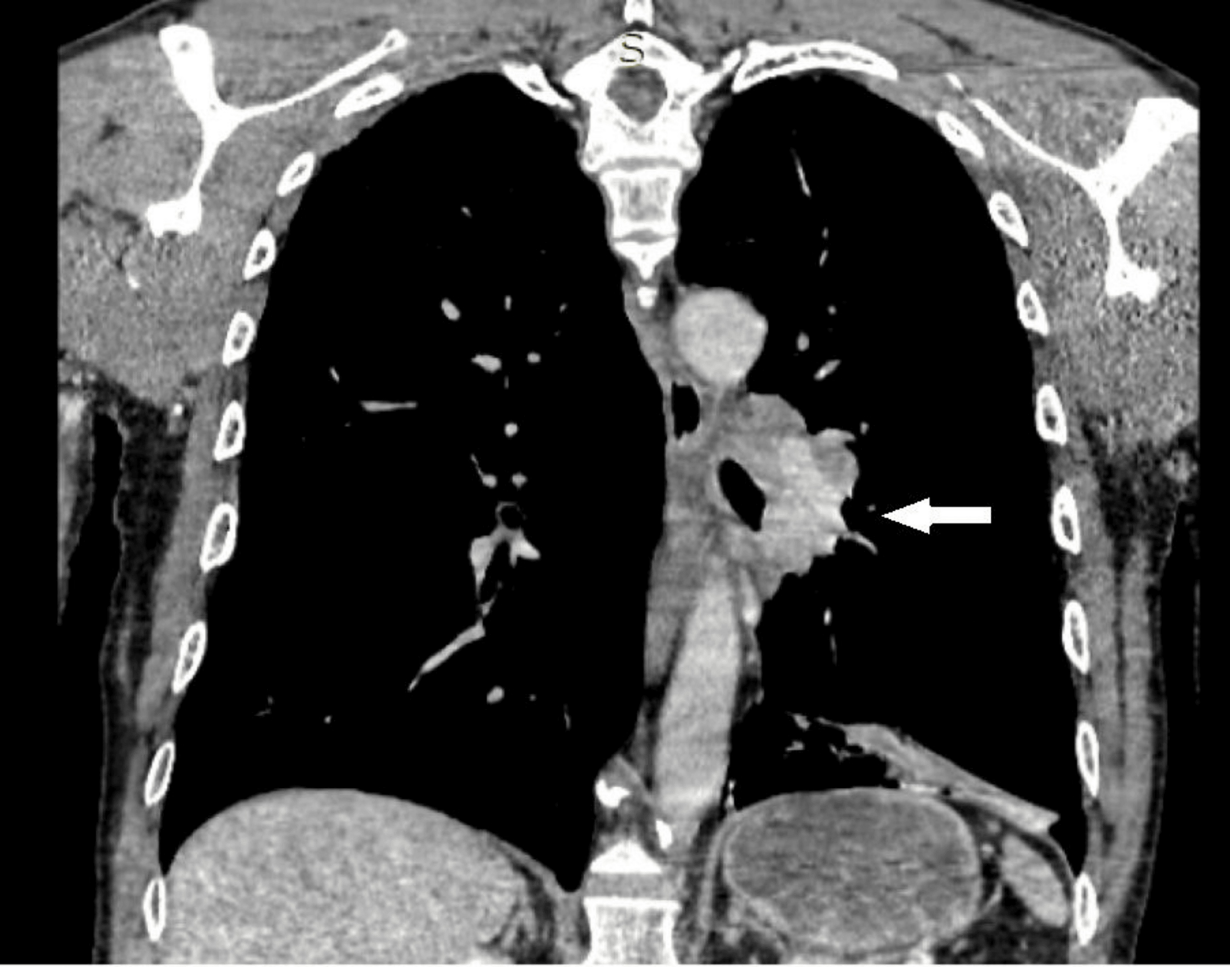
The coronal section of the preoperative thoracic CT scan showing tumor relationship to the left hilum. CT: computed tomography
